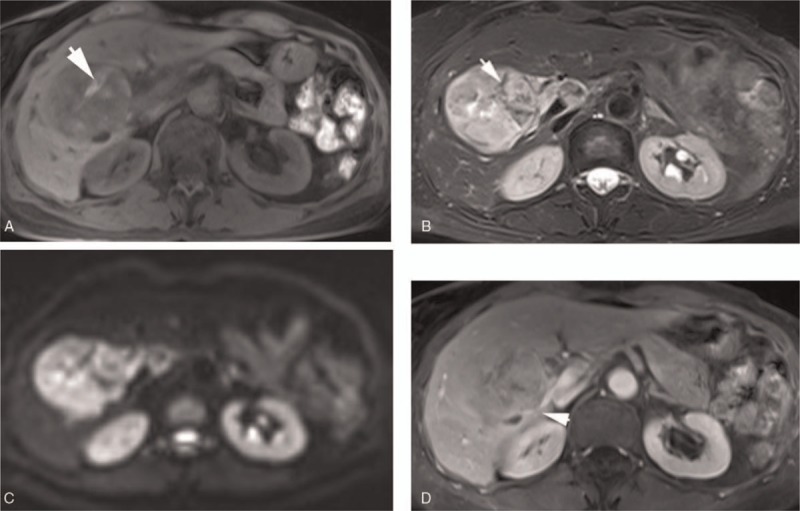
Magnetic resonance imaging revealed a heterogeneous mass in the hepatic hilar region. The mass was lobular and demonstrated expansive growth. (A) The mass displayed uneven low-signal intensity on T1-weighted imaging (T1WI) and (B) slightly high-signal intensity on T2-weighted imaging (T2WI). The strip in the tumor exhibited high-signal intensity on T1WI and low-signal intensity on T2WI (arrow), suggesting the existence of melanin. (C) Diffusion-weighted imaging revealed a restricted diffusion change, and (D) the tumor was unevenly enhanced after enhancement. Irregular liquefactive necrosis was discerned in the tumor. The tumor compressed the intrahepatic bile duct and caused dilatation and also invaded the right branch of the portal vein (arrow).
